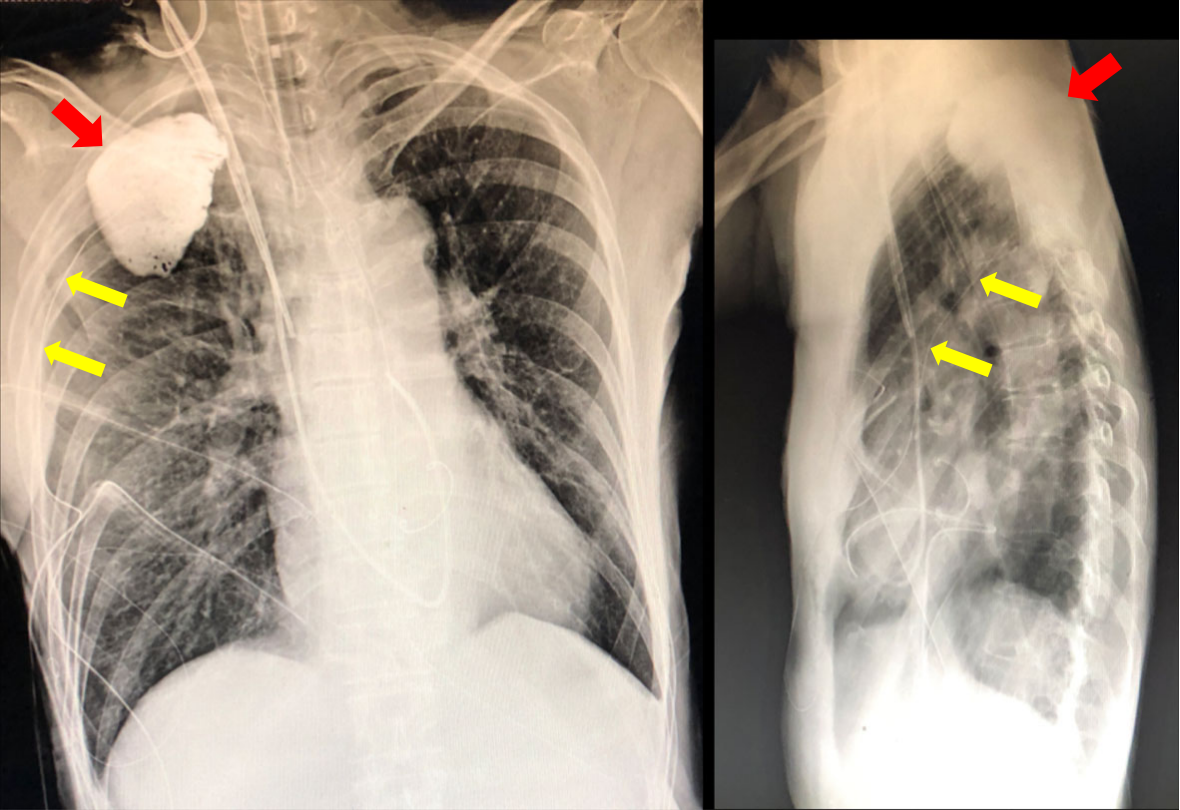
Frontal and lateral chest radiograph images showing the spread of contrast medium. The contrast medium was localized across the 3rd to the 5th intercostal area in the posterior chest. Yellow arrows indicate the inserted catheter, and red arrows indicate the administered contrast medium
